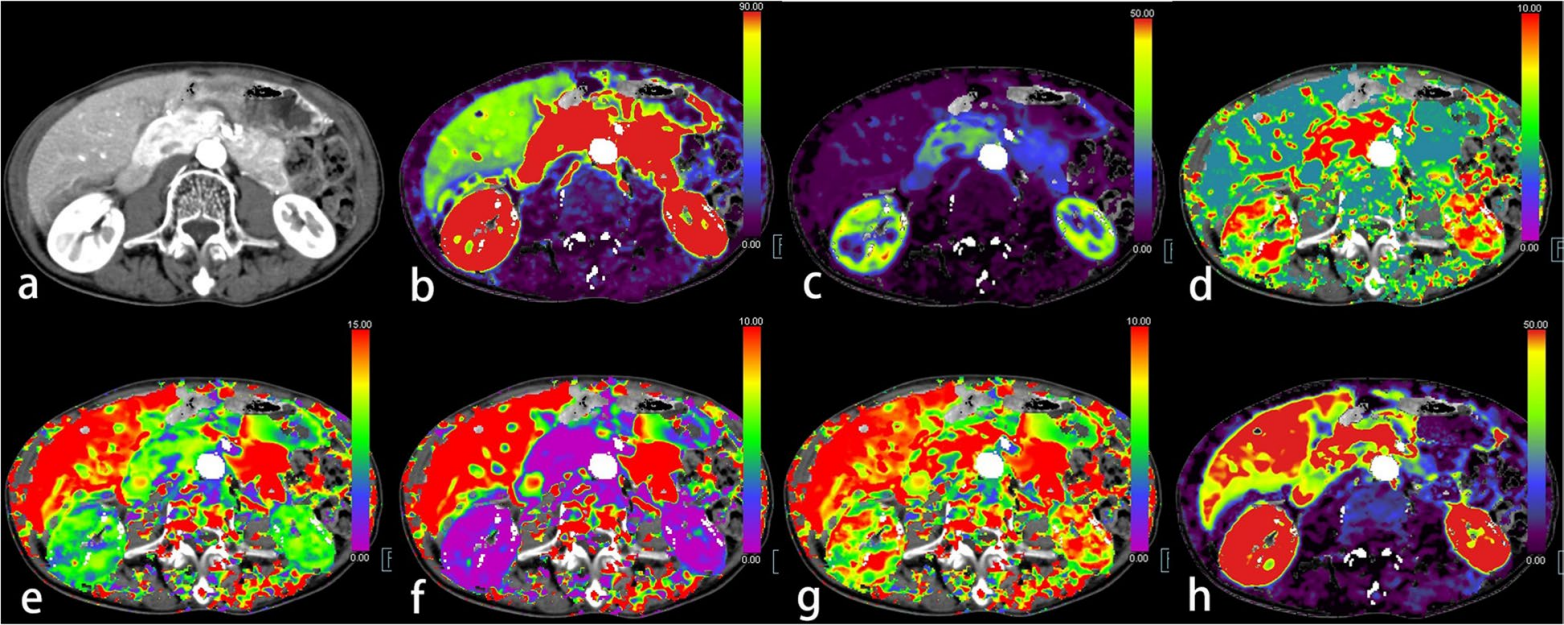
A 66-year-old male with osteoporosis, low muscular mass, and high-grade sigmoid rectal cancer (T_4a_N_1_M_1a_). a Axial abdominal contrast-enhanced CT image located at the central level of the vertebral body of lumbar 3(L3). b The blood flow (BF) map of the CT perfusion (CTP). c The blood volume (BV) map. d The mean transit time (MTT) map. e The time to drain (TTD) map. f The time to start (TTS) map. g The time to maximum (T_max_) map. h The flow extraction product (FE) map
